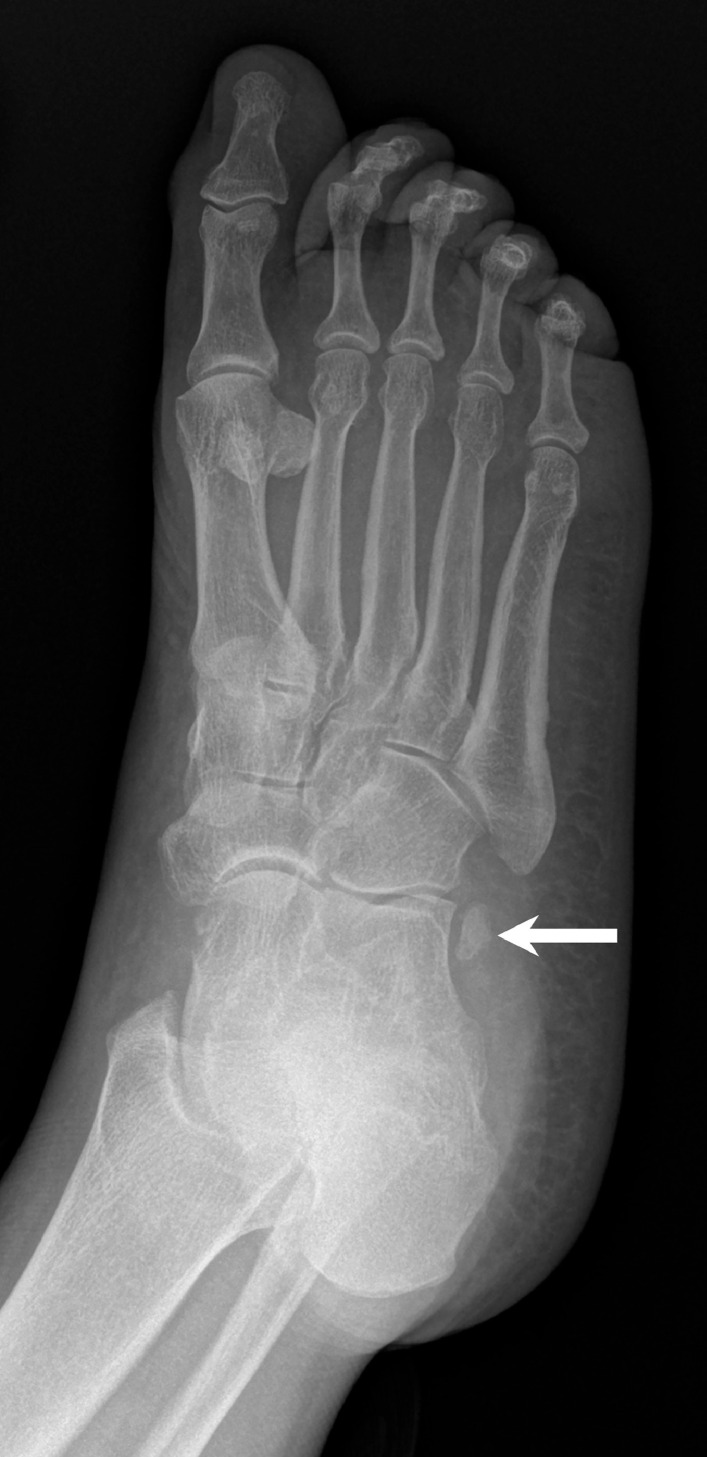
Oblique radiograph of the right foot at the time of initial presentation demonstrates an os peroneum at the expected level of the calcaneocuboid joint (arrow).
